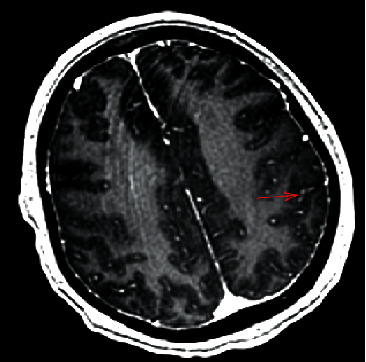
MRI brain with IV contrast suggesting sulcal enhancement of the gyrus representing underlying leptomeningeal disease.
